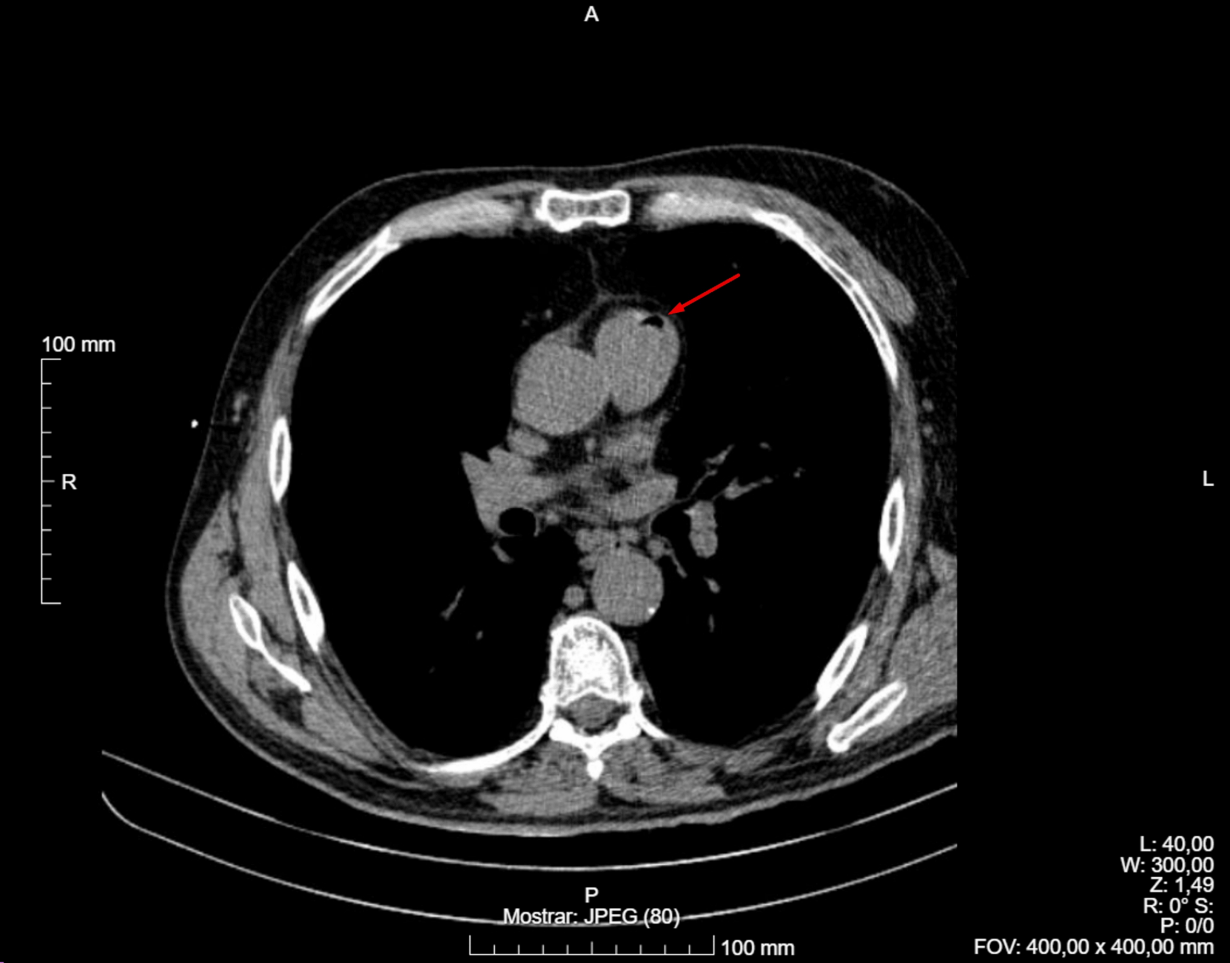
Control CT scan showing air embolism in the left ventricle.
